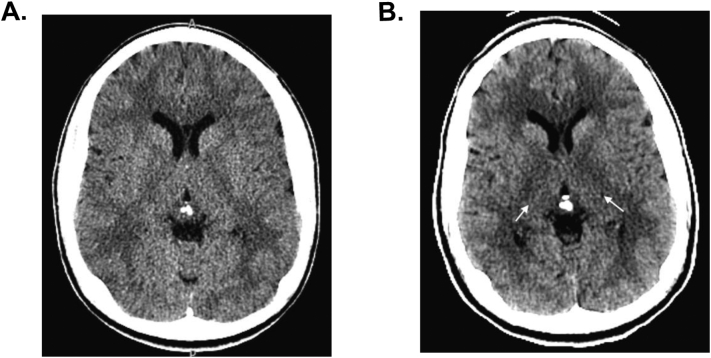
CT scan of the patient's head. A) Baseline axial CT image through the cerebral deep gray nuclei shows symmetric, normal gray-white matter distinction without focal cerebral lesions. B) Axial CT image obtained during the recent hospitalization. Image through cerebral deep gray nuclei shows subtle hypodensity infiltrating the thalami, internal capsules, and adjacent globi pallidi (arrows) consistent with edema in the setting of metabolic decompensation.
